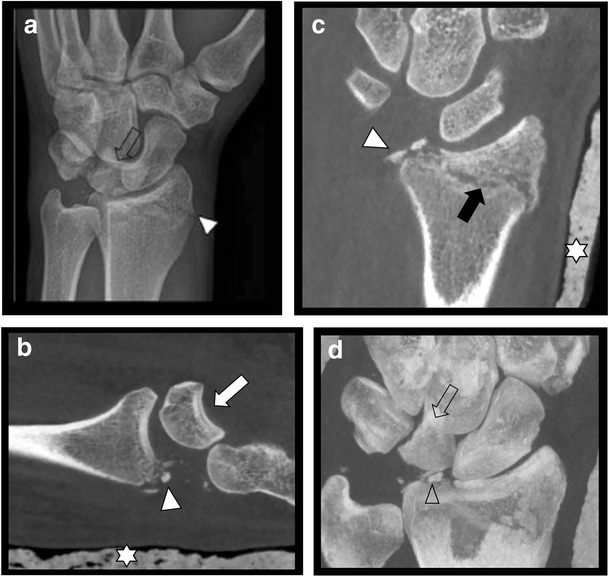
Complex fracture of the dorsal side of the styloid process of the radius with intra-articular involvement, multiple intra-articular fracture fragments and perilunate dislocation. a. CR (oblique view) shows a fracture of the distal radius (arrowhead) and perilunate dislocation (arrow). b. Sagittal CBCT reformatted image after immobilization and casting (stars) shows residual perilunate dislocation (white arrow) with dorsal displacement of the distal carpal row and additional fracture fragments (arrowhead). c. Coronal CBCT reformatted image after immobilization and casting (stars) demonstrates distal radius fracture (black arrow). The degree of communition and additional fracture fragments better seen than on plain films (arrowhead). d. 3-D reconstruction may be useful for evaluation of the displacement of the carpal bones (open arrow) and additional fracture fragments (open arrowhead)
